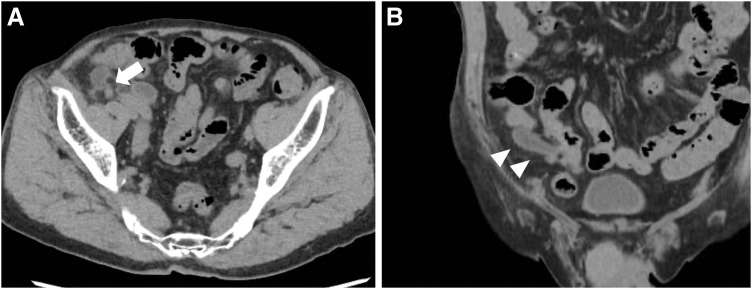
Follow-up CT before IA. (A, B) Follow-up CT showing resolution of the acute periappendiceal inflammation. Discontinuity between the appendiceal base and the distal segment (arrow) suggests transection. The distal segment appears adherent to the adjacent small intestine, and the distal tip is enlarged (arrowhead). IA, interval appendectomy
